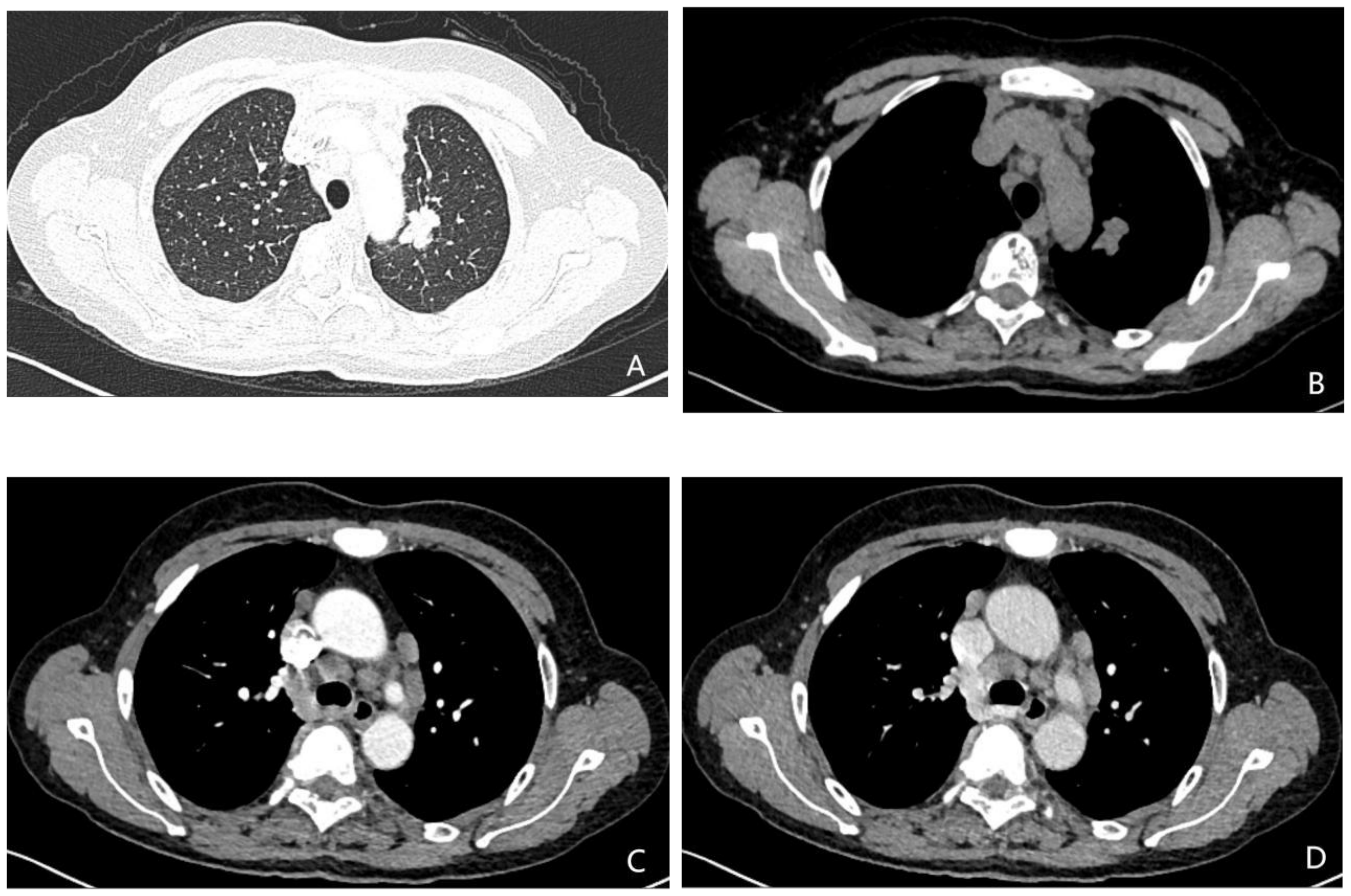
(A-D) A 58-year-old female patient with lung adenocarcinoma, the genetic test result was EGFR 19Del mutation (+). (A-B) Unenhanced CT demonstrates an irregular soft-tissue density shadow in the left upper lobe, measuring 25.3 mm in maximum diameter with lobulated margins. (C, D) Contrast-enhanced CT shows multiple enlarged lymph nodes adjacent to the trachea and aorta during arterial and venous phases.
